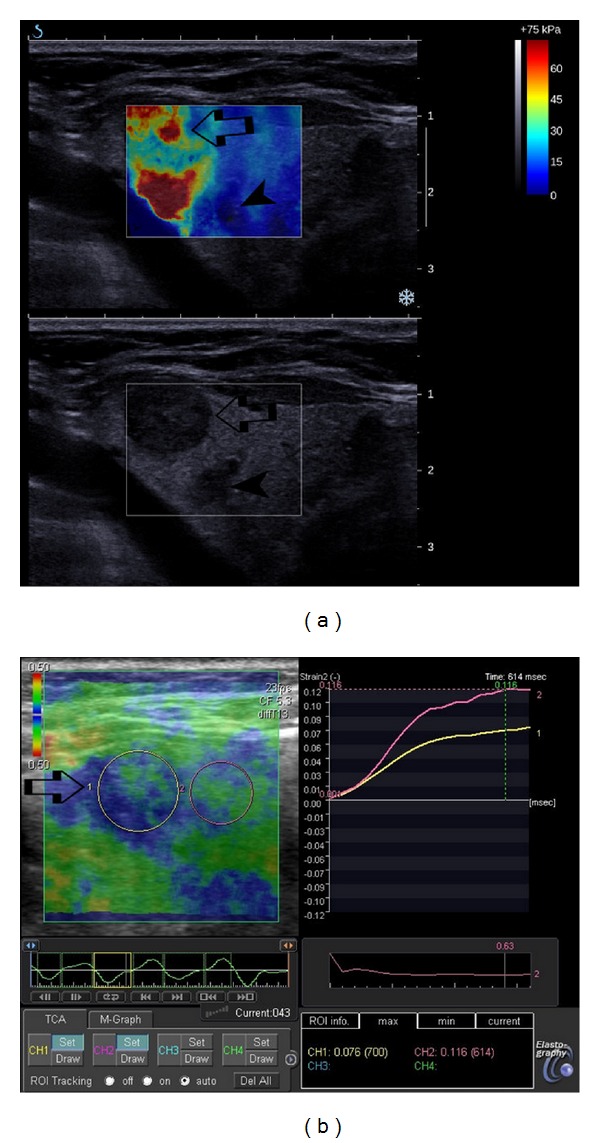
Diffuse sclerosing papillary carcinoma of the left lobe of the thyroid gland (arrow) in patient with multinodular goiter and multiple colloid nodules (arrow head). (a) on B-mode ultrasound (lower image), the cancer is less suspicious than some of the colloid nodules; however, on supersonic shear wave elastography (upper image) the nodule presents areas of high stiffness (over 75 kPa) indicating malignant lesion. The colloid nodule presents as a lesion with low stiffness. (b) on strain elastography, the carcinoma qualitatively and quantitatively presents features of soft lesion falsely indicating benign nature of the lesion.
